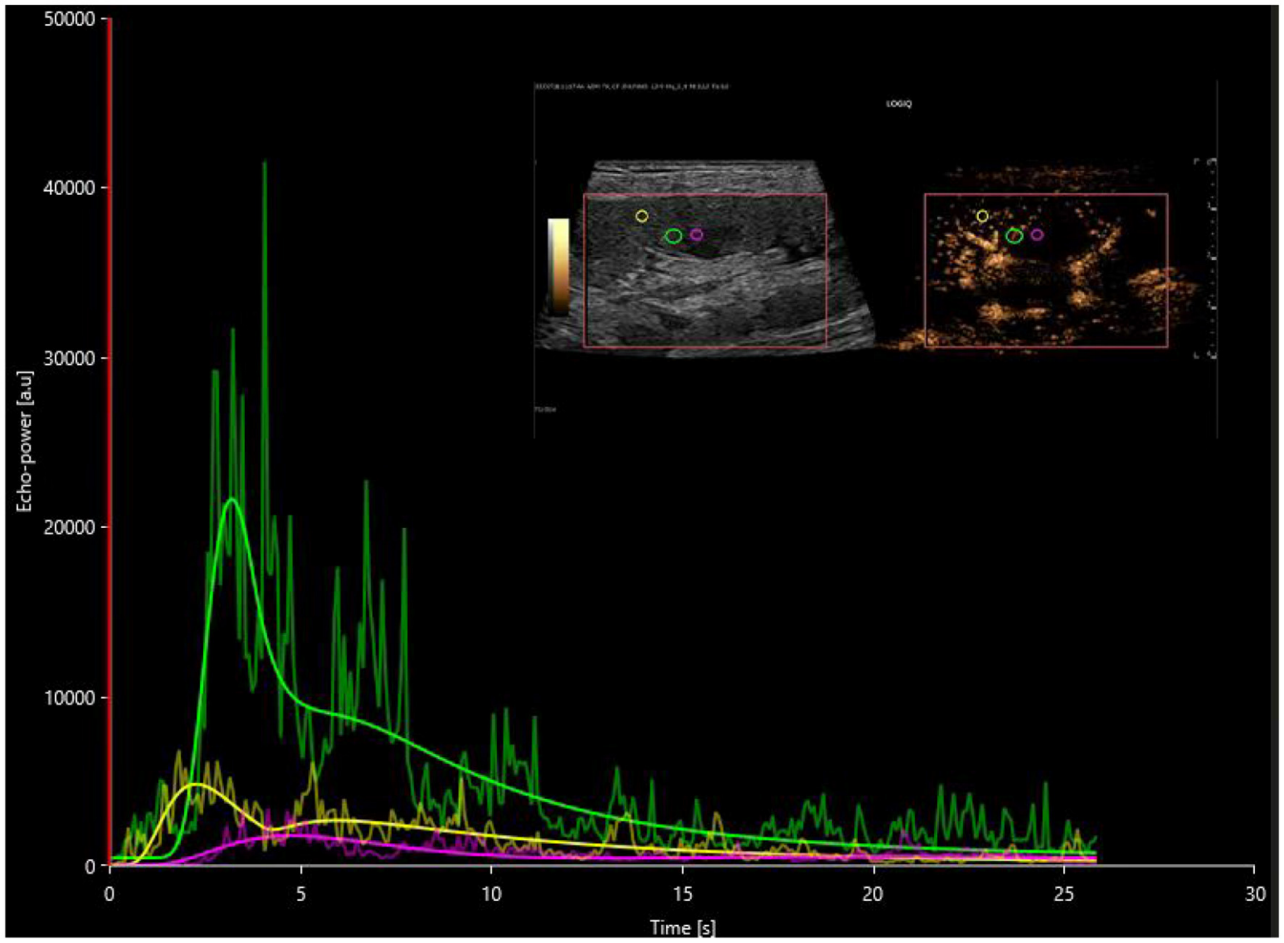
Postoperative time-intensity curves of contrast-enhanced ultrasound in the AR group, with regions of interest (ROIs) highlighted for the cortex (yellow), medulla (pink), and a combination of partial cortex and medulla under the large capsule (green).
